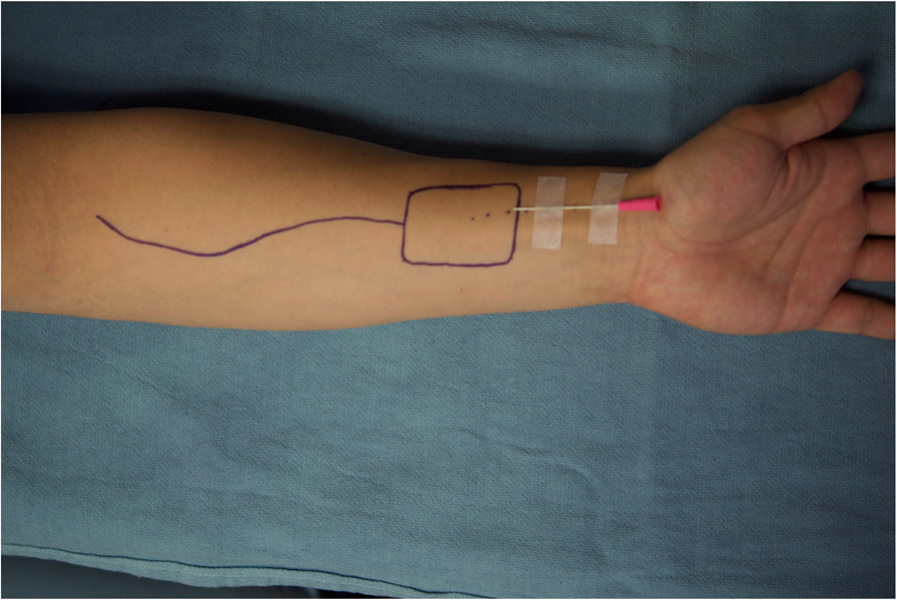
Redesigning a more proximal radial forearm free flap avoids the area of likely vessel trauma from an intra-arterial cannula. In this image, a standard intra-arterial cannula is positioned overlying the distal radial artery. The exact length of stenosis can be confirmed with Doppler ultrasonography
